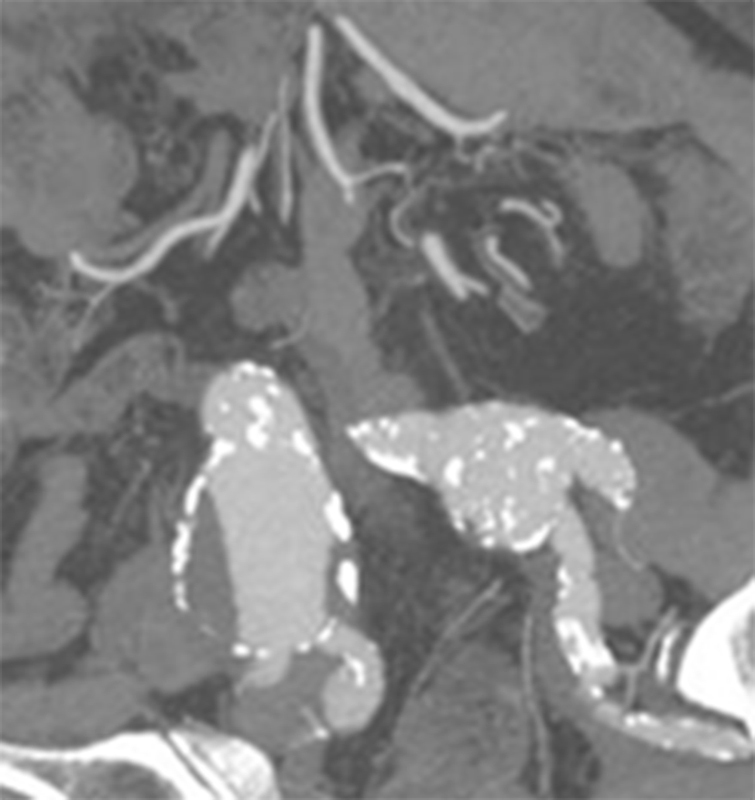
Computed tomography angiography showing the remarkable distance between the orifices of the internal iliac artery and external iliac artery in both sides.
